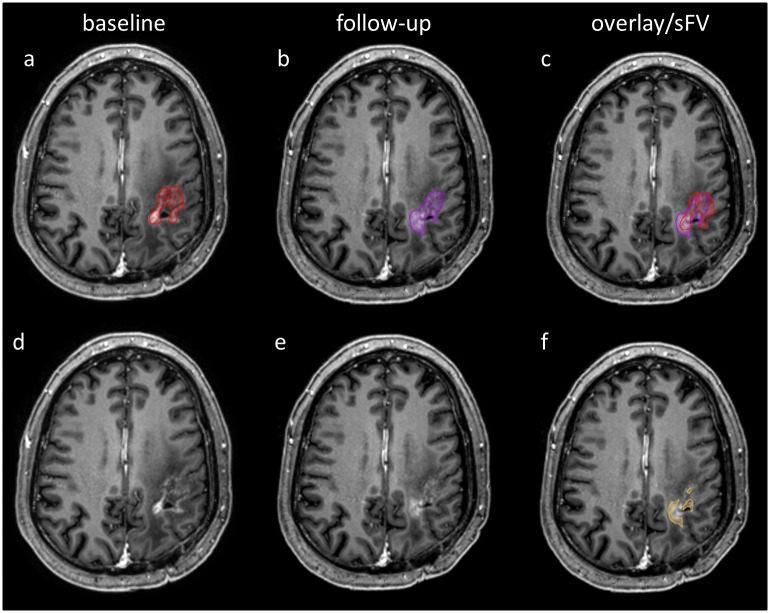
Examples of contrast-enhanced MPRage segmentations and regional, segmentation-based subtractions. Axial MPRage slices of the baseline MRI (a, d) and the follow-up MRI (b, c, e, f) of a patient with GB are shown. Segmentation of baseline CV (a, red), follow-up CV (b, purple) and an overlay image of both segmentations (c) is presented. Baseline MPRage image (d) and follow-up MPRage image (e) are displayed without segmentations. The regional, segmentation-based subtraction of CV (sCV) is also shown (f, yellow).
